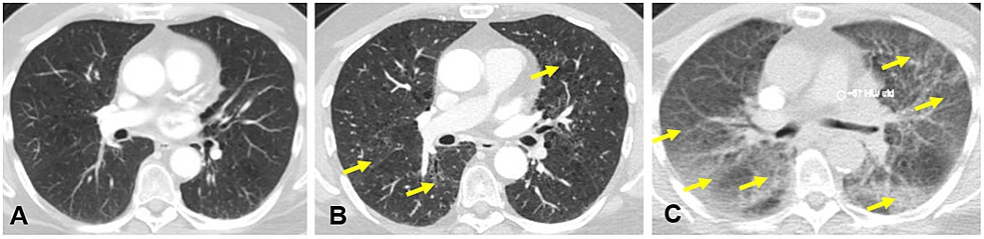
Comparison of computerized tomography (CT) thorax images before and after initiation of consolidation therapy (A) CT thorax with contrast 10 days prior to durvalumab initiation. (B) CT thorax with contrast showing new opacities (arrows) following the patient’s development of dyspnea. (C) CT angiogram on admission for this case report, showing worsened and new diffuse opacities (arrows) following antibiotic and steroids course, prior to infliximab.
